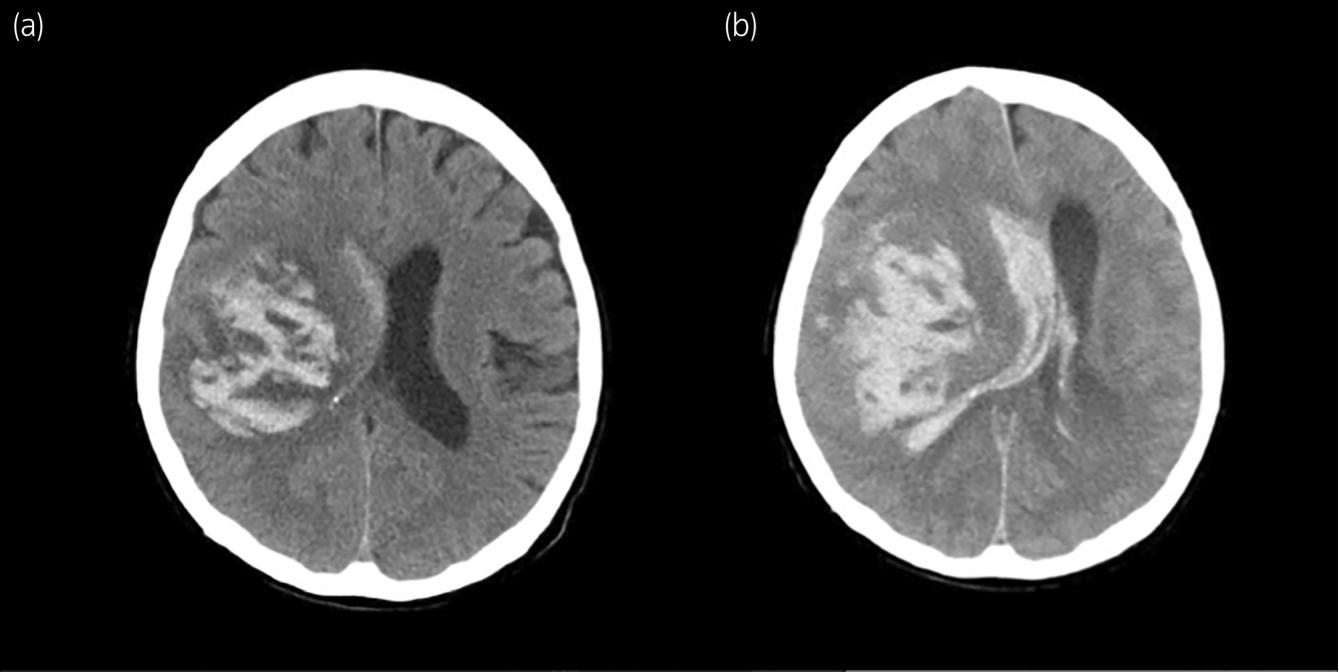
CT images of the cerebral hemorrhage. CT scans of the head revealed a cerebral hemorrhage appearing to originate from a metastatic lesion in the right insular cortex. The left image was taken immediately after the hemorrhage (a), and the right image was taken 6 h after the hemorrhage (b).
